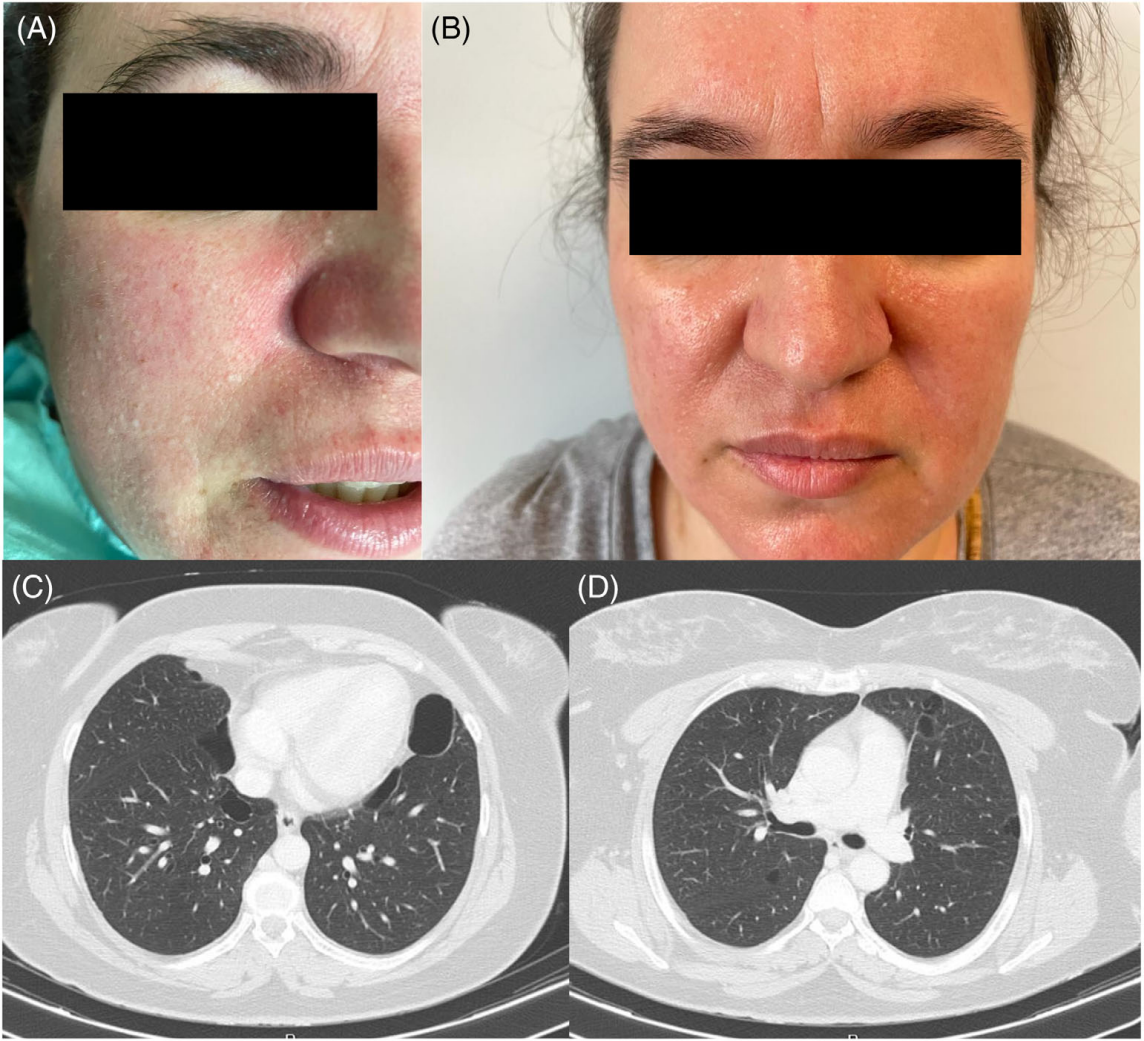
(A, B) Multiple whitish dome‐shaped papules visible in the nose and paranasal area. Biopsy demonstrated fibrofolliculomas. (C, D) Chest computed tomography scan axial cut showed thin walled, round and ovoid pulmonary cysts predominanting in the lower‐medial zone of both lungs
